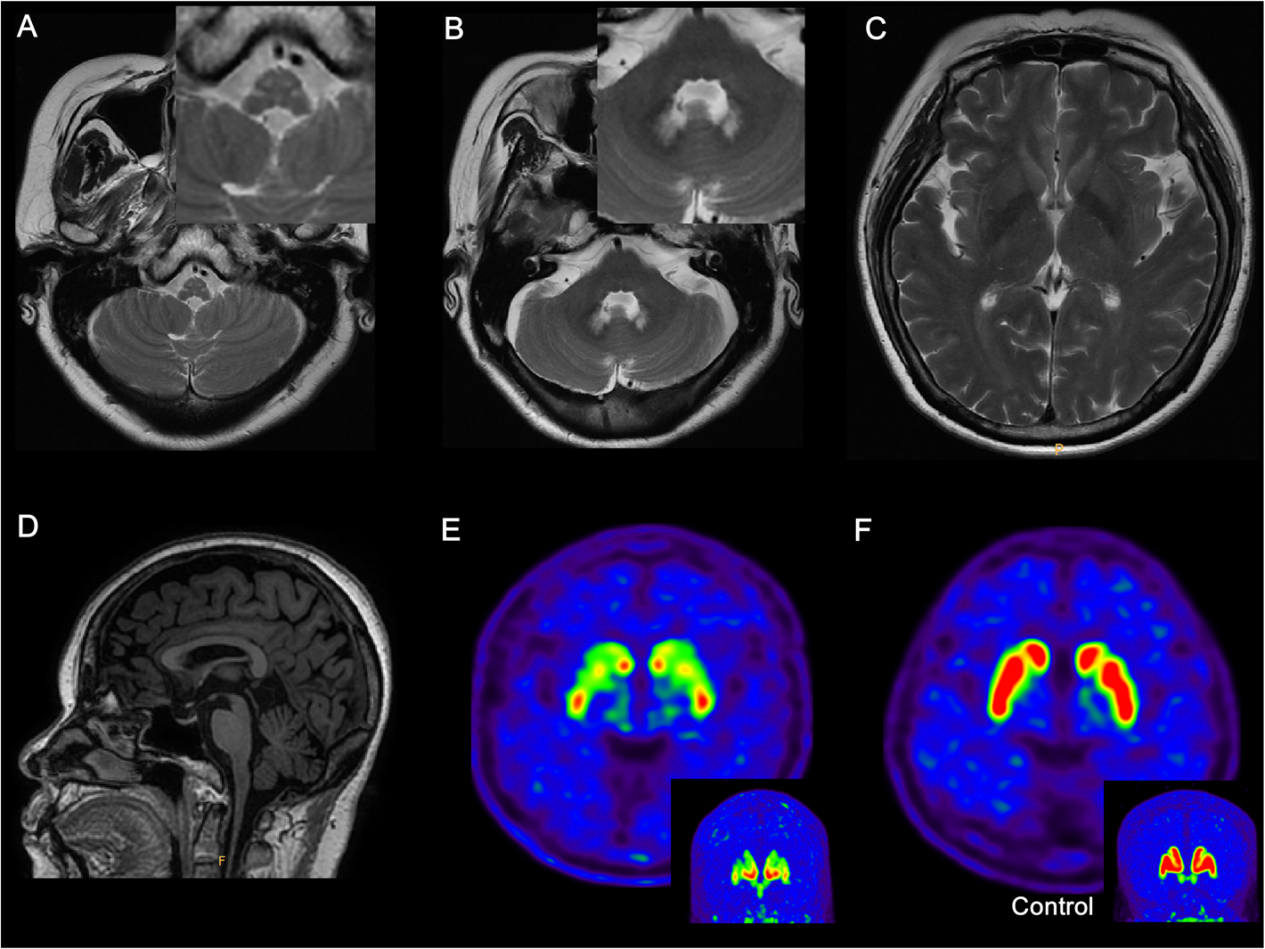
Brain MRI and FP-CIT PET imaging of the patient. a, b Brain MRI with T2-weighted axial images show typical bilateral high signal intensity at both medulla oblongata and cerebellar dentate nuclei. c No major lesions in the basal ganglia and periventricular white matter. d A T1-weighted sagittal image shows tadpole shape cervicomedullary atrophy. e 3-dimensional [18F] FP-CIT PET shows severely decreased dopaminergic uptake in bilateral putamen and caudate nucleus with a rostrocaudal gradient. f FP-CIT PET in control shows normal dopaminergic uptake in bilateral putamen and caudate nucleus
